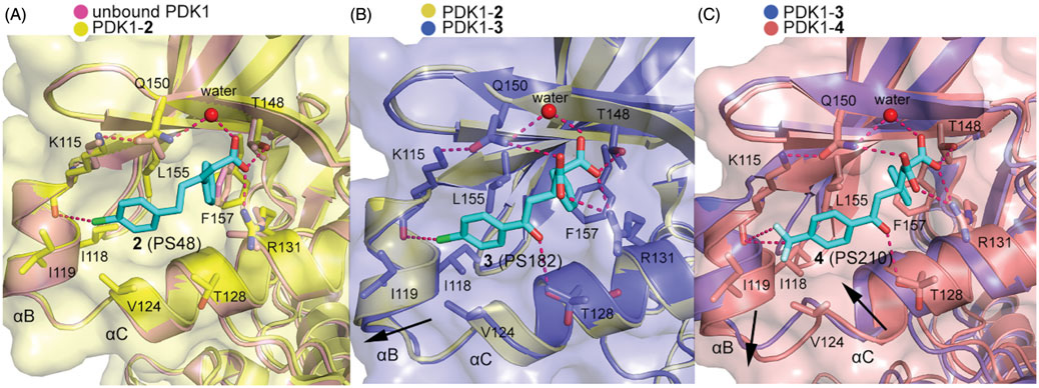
(A) Comparison of the X-ray structure of unbound PDK1 (pink; PDB ID: 3HRC) with the X-ray structure of PDK1-2 complex (pale yellow; PDB ID: 3HRF). (B) Comparison of the X-ray structure of PDK1-2 complex with the X-ray structure of PDK1-3 complex (slate; PDB ID: 4AW0). (C) Comparison of the X-ray structure of PDK1-3 with the X-ray structure of PDK1-4 complex (salmon; PDB ID: 4AW1).
